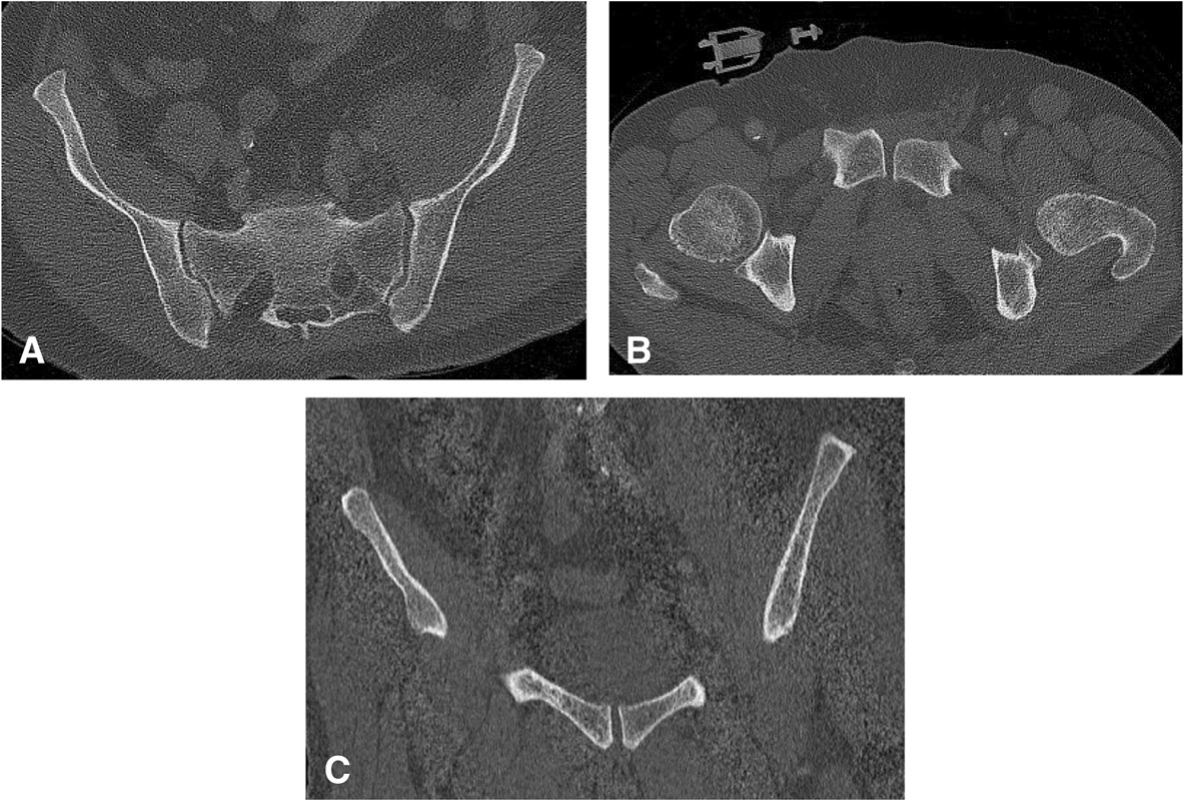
Initial computed tomography scans. CT scans of the pelvis with external pelvic stabilization (SAM Pelvic Sling™ II) in place (b). Slightly disproportionate left iliosacral joint, yet no bony injuries are visible (a). The pubic symphysis appears normal in width and configuration (b, c)
